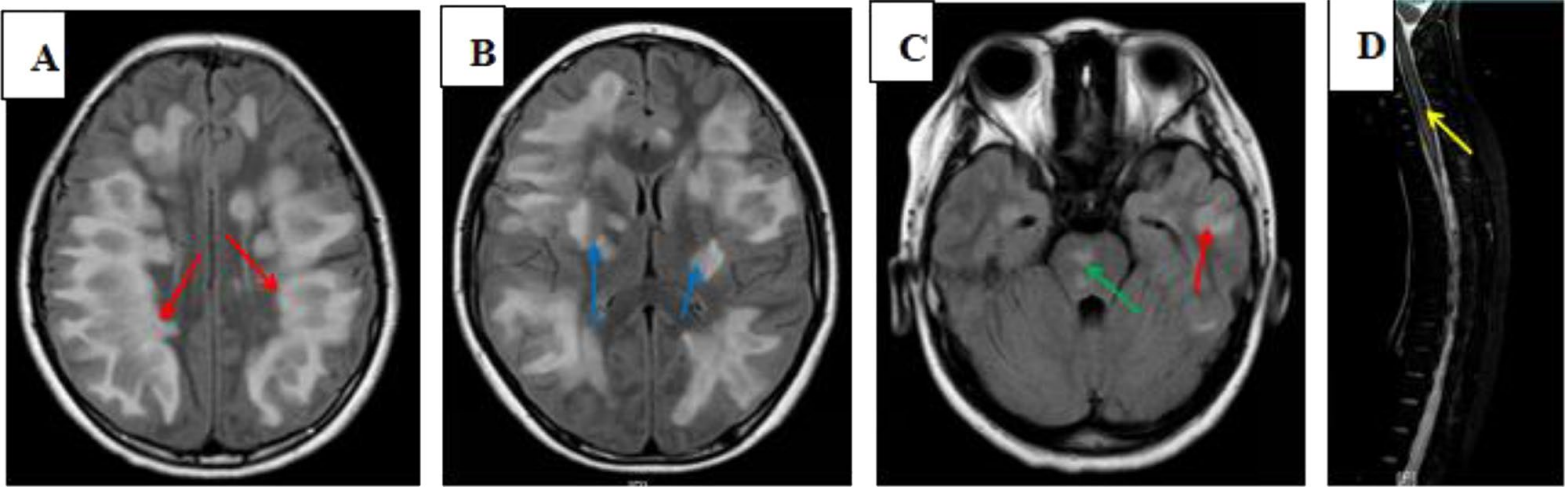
Typical brain and spinal T2-weighted MRI were recorded in 3-year-old patient with ADEM: widespread, blurred (arrowhead), large lesions involving both hemispheres (A), the thalamus and brainstem (B and C), and longitudinally extensive myelitis (D)
